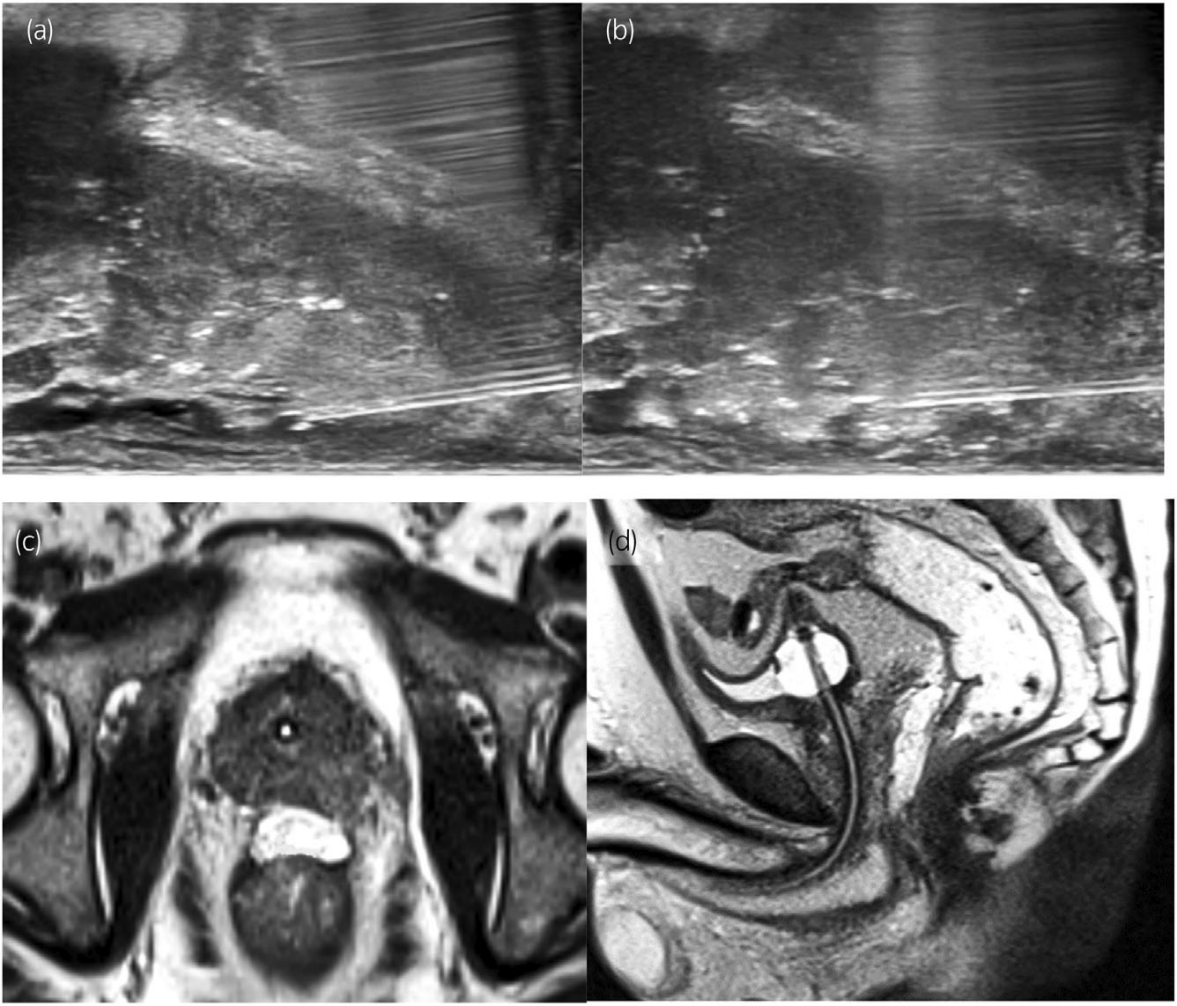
Ultrasound imaging during procedure (a, b) shows no abnormalities. T2‐MRI images from the following day (c, d) indicate successful gel placement.
